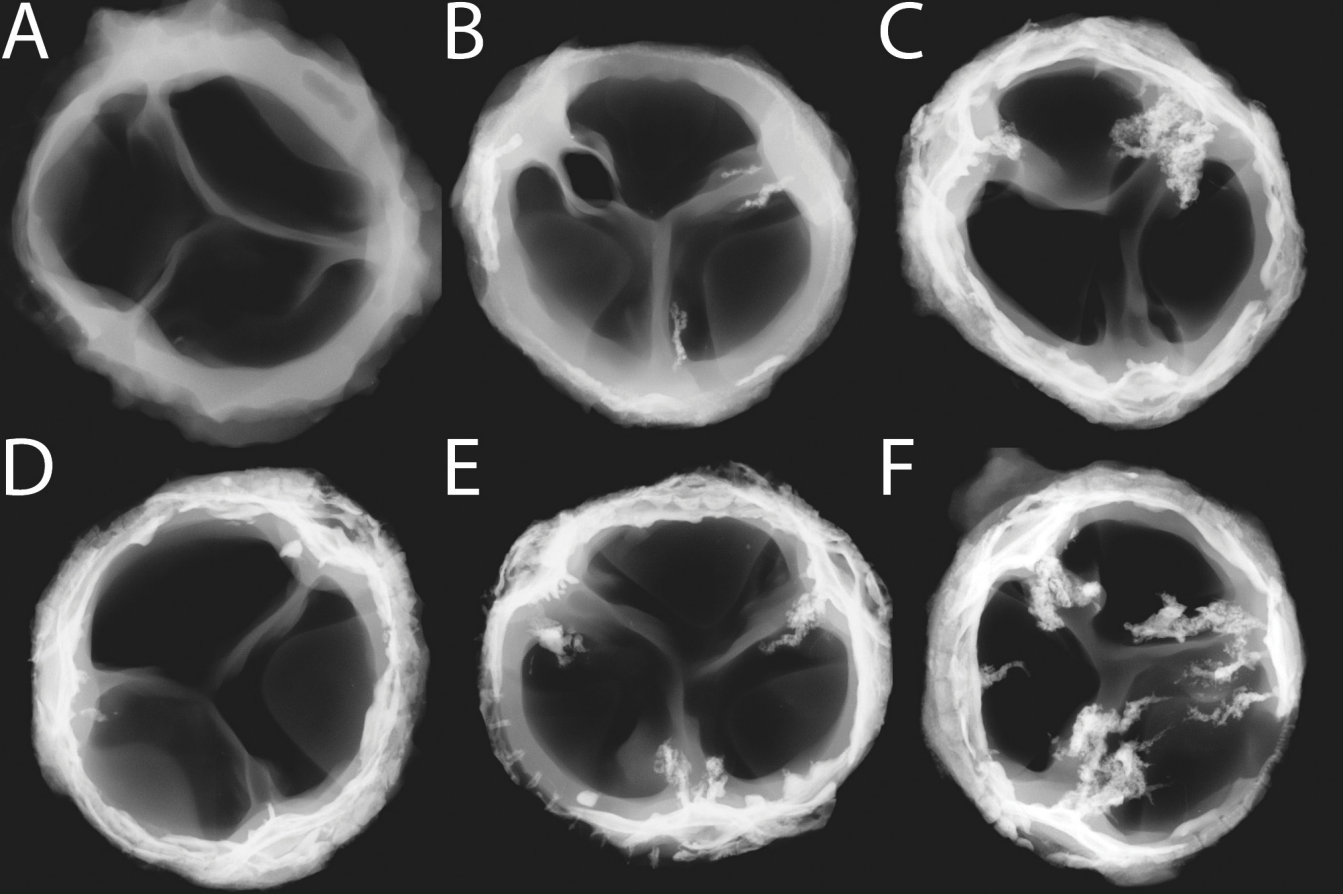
High resolution X-ray after the sewing ring and stent have been removed. A) Not implanted control valve without any calcification. B-D) Minor calcific depositions can be seen at two of the commissures. E-F) Various degrees of calcification involving all three commissures and parts of the belly area.
